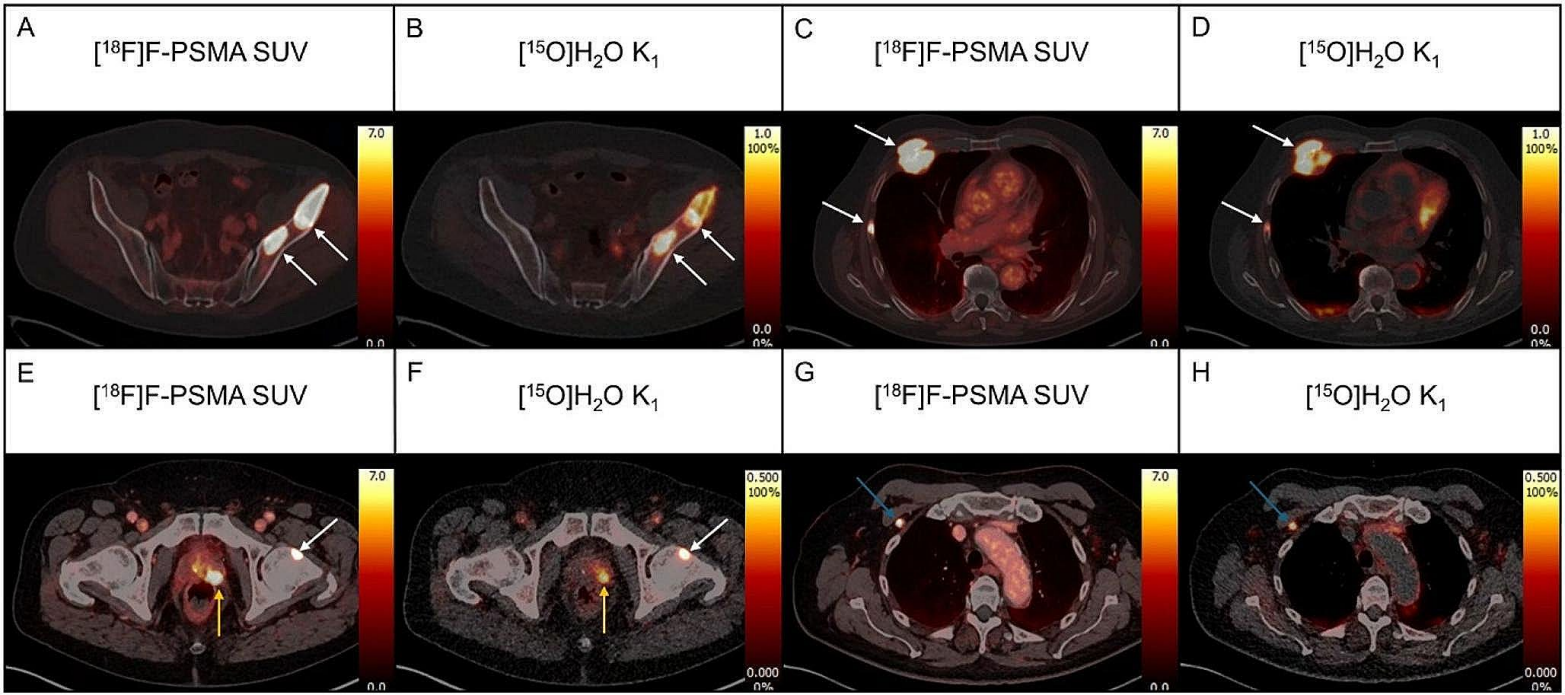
Transaxial fused [^18^F]PSMA-1007 SUV PET/CT images for tumour location (A, C, E, and G, arrows) and parametric trans axial fused [^15^O]H_2_O K_1_ images (mL/min/mL) (B, D, F, and H) showing highly increased perfusion in bone metastases (B, D and F, white arrows), primary tumour (F, orange arrow) and lymph node metastasis (H, blue arrow)
